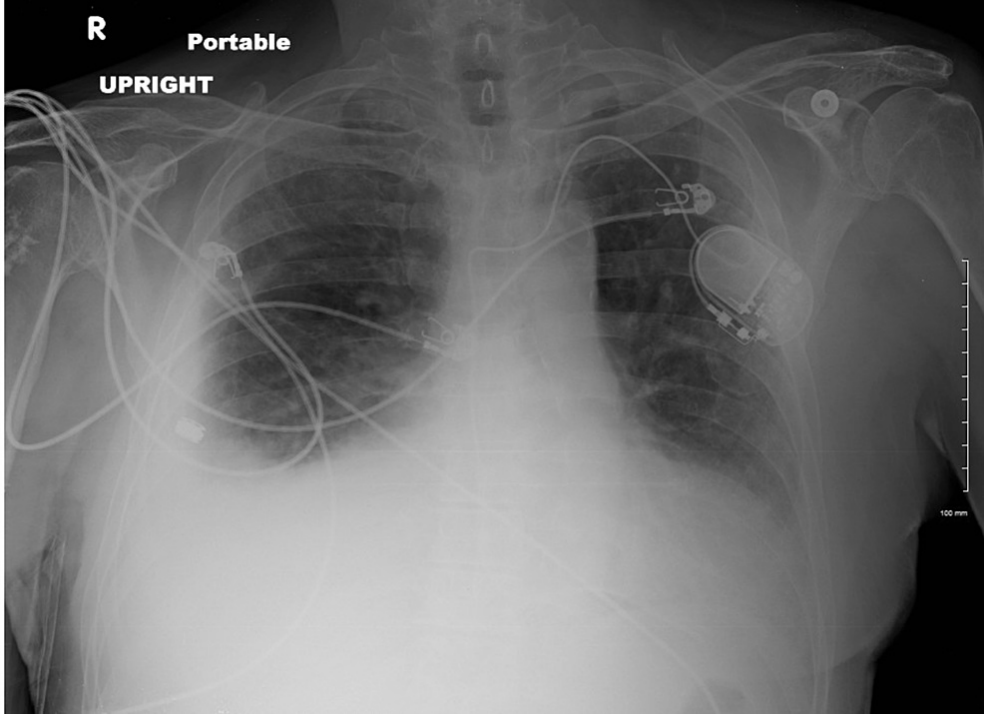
Chest X-ray (1st Admission)
